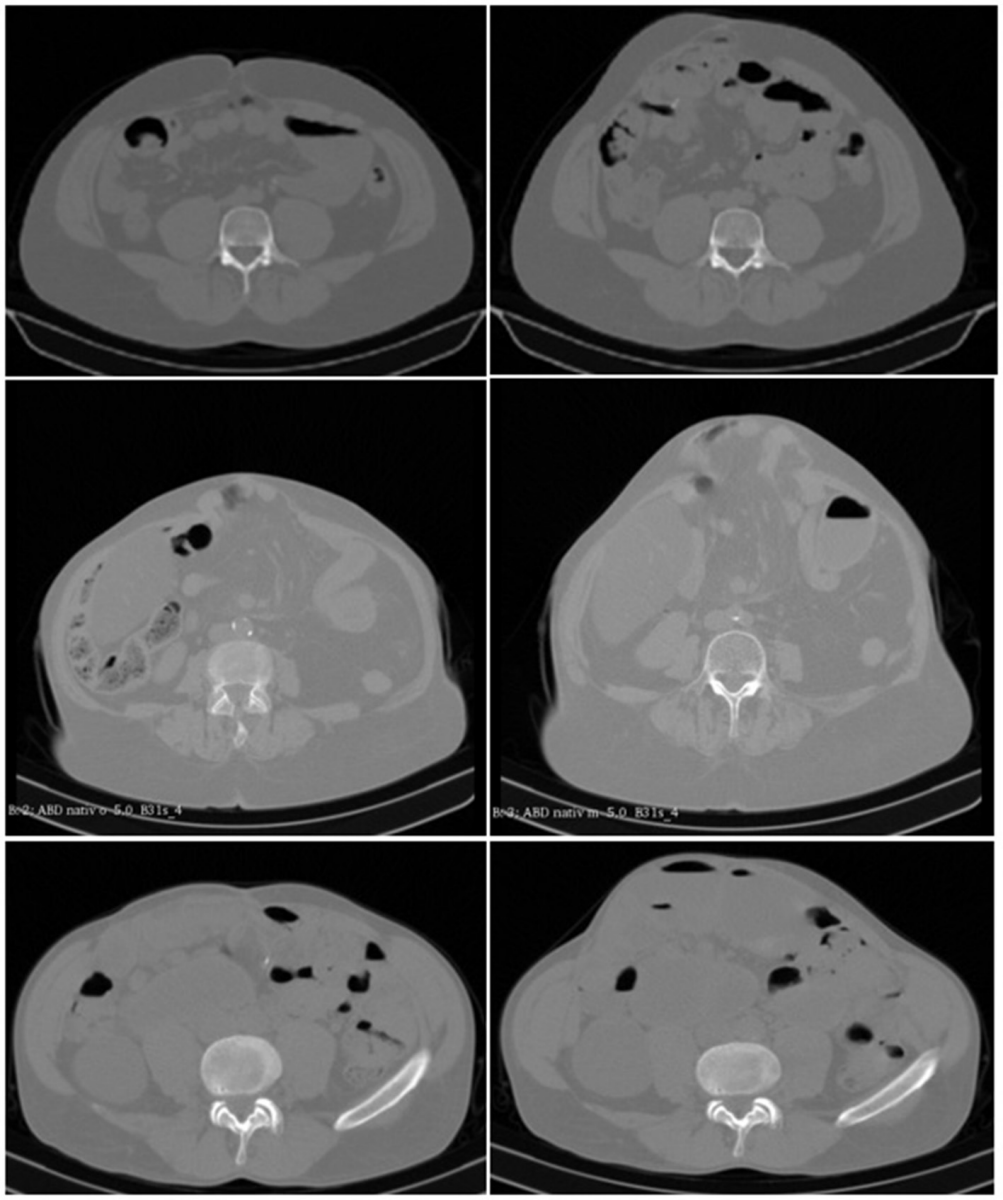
(Top) CT scans of the abdomen without contrast medium of a 35-year-old male with an incisional hernia after liver transplantation at rest (Left) and during Valsalva's maneuver (Right). It can be noted that the lateral musculature contracts, and the abdominal contents bulge forward with a distension of the hernia opening by about 30%. (Middle) CT scans of the abdomen without a contrast agent of a 73-year-old woman with an incisional hernia after pancreatic resection at rest (Left) and during Valsalva's maneuver (Right). It can be seen that the anterior abdominal wall bulges forward, and the hernia sack increases in size without enlargement of the hernia opening. (Bottom) CT scans of the abdomen without added contrast of a 62-year-old man with an incisional hernia after laparostoma and short bowel syndrome resulting from multiple intestinal fistulae after an ileus. The functional state at rest (Left) and during Valsalva's maneuver (Right) corresponds to the two upper rows. It can be recognized that the left lateral musculature is displaced by the abdomen bulging forward opening the hernia base by about 50%. (we acknowledge the help of Samuel Voss in the selection of scans in the upper and lower row).
